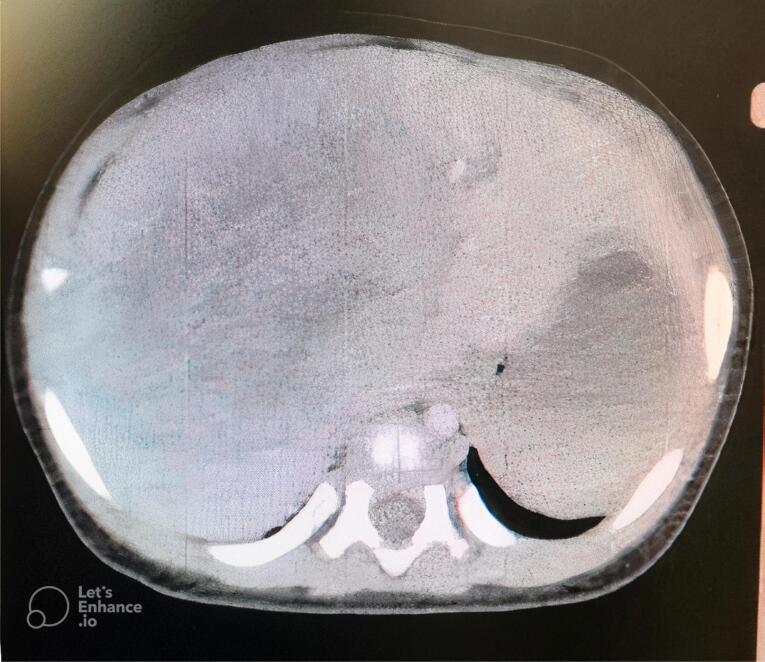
After confirmation of the diagnosis of primary hepatic NB, the patient was started on a chemotherapy regimen. After 4 sessions of chemotherapy with an interval of 21 days (using vincristine, doxorubicin, and cyclophosphamide #3/cisplatin and etoposide #1), an ultrasound was performed for the patient, representing that the mass size had decreased to 55 × 36 mm.
